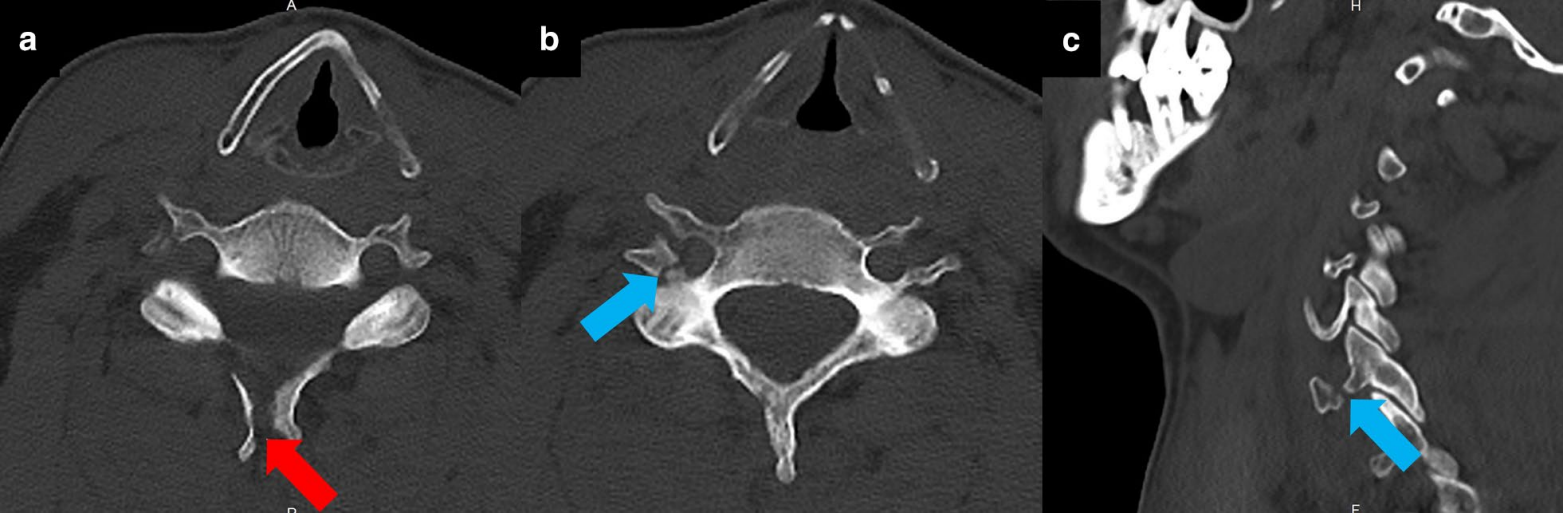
Diagnostic error. A 48-year-old male patient was admitted to the ER with head trauma after falling from a height of 2 m. The on-call radiologist reported a displaced fracture at the spinous process of the C6 vertebra (red arrow, a). Another displaced fracture at the posterior and lateral wall of the right transverse foramen of the C6 vertebra was missed (blue arrows, b, c). Emergency physicians were informed about the fractures after a second review of CT images. CT angiography performed to rule out vertebral artery injury, revealed no vascular injury
